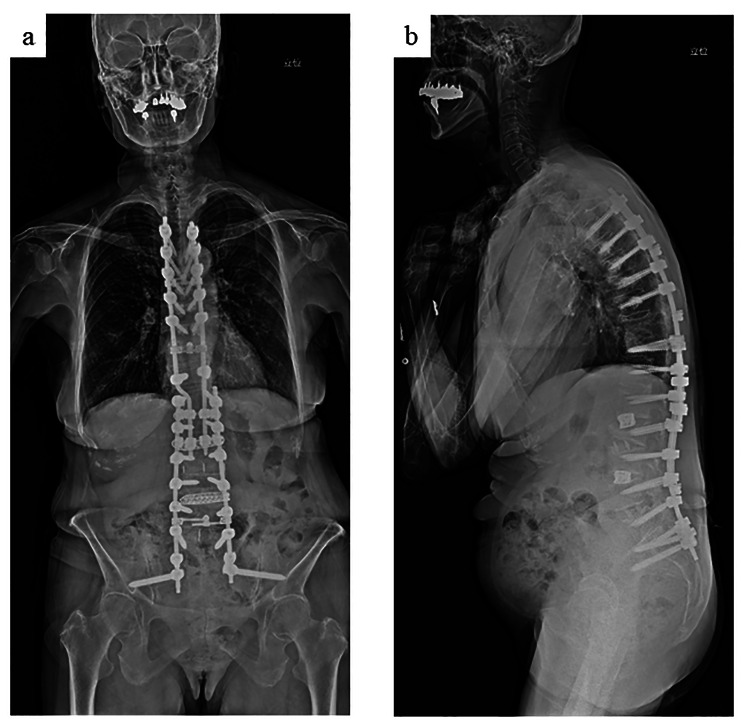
Standing X-ray after revision surgery. We removed the loosened pedicle screws at T10 and extended the fusion surgery to T5
